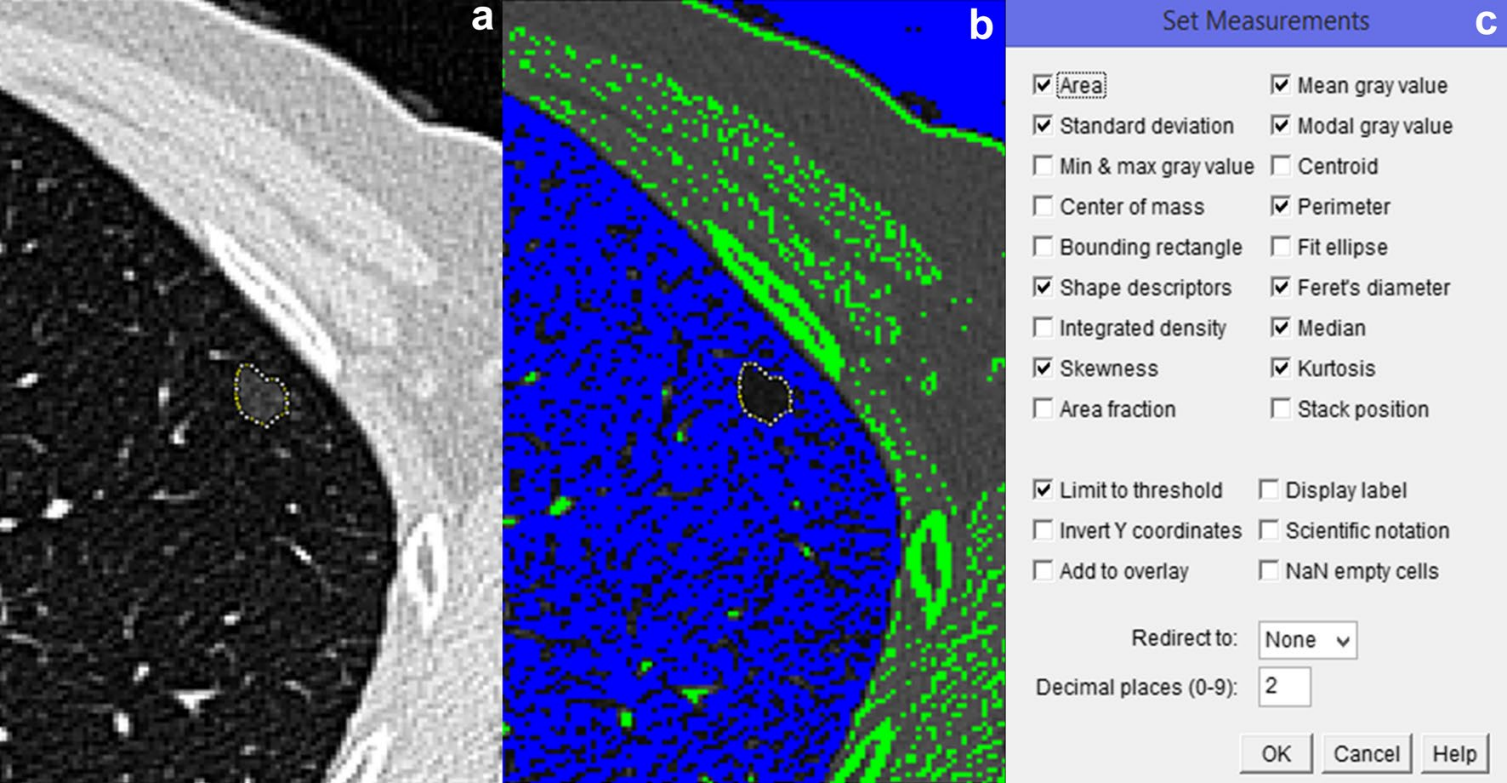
Images showing a summary of the steps of software-based analysis using ImageJ in a nonsolid nodule (NSN): a polygonal region of interest outlining the NSN contours, b threshold image obtained by applying an attenuation value of − 800 HU suitable for NSN segmentation, c list of quantitative CT features selected for NSN analysis
